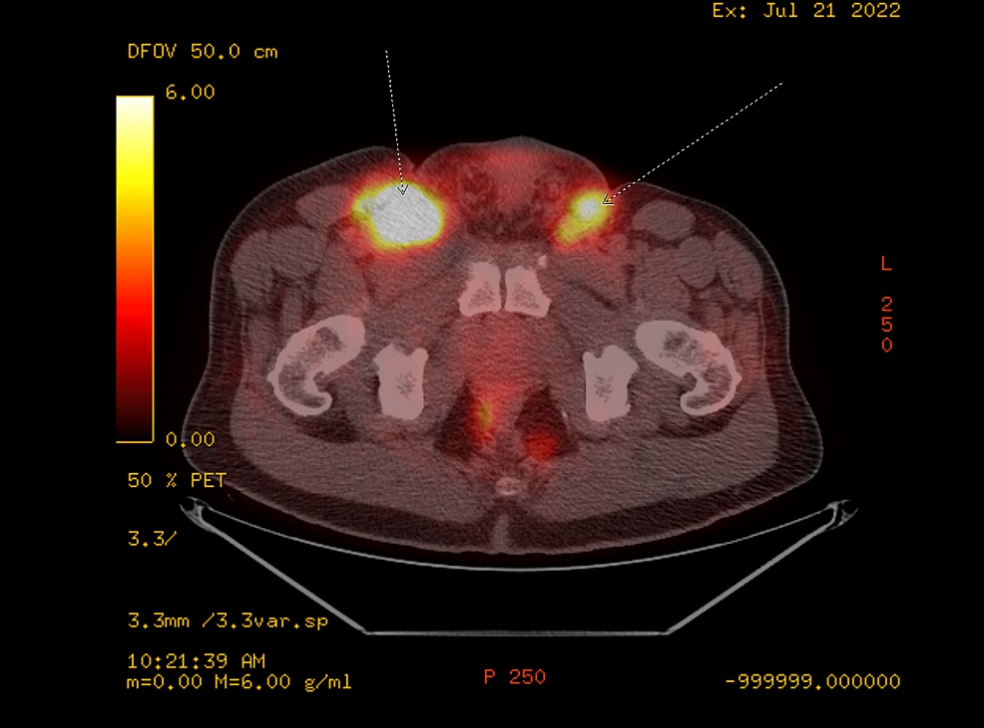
Pre-chemotherapy PET/CT demonstrating bilateral inguinal involvement Arrows pointing at areas of hypermetabolic activity in metastatic inguinal lymph nodes
